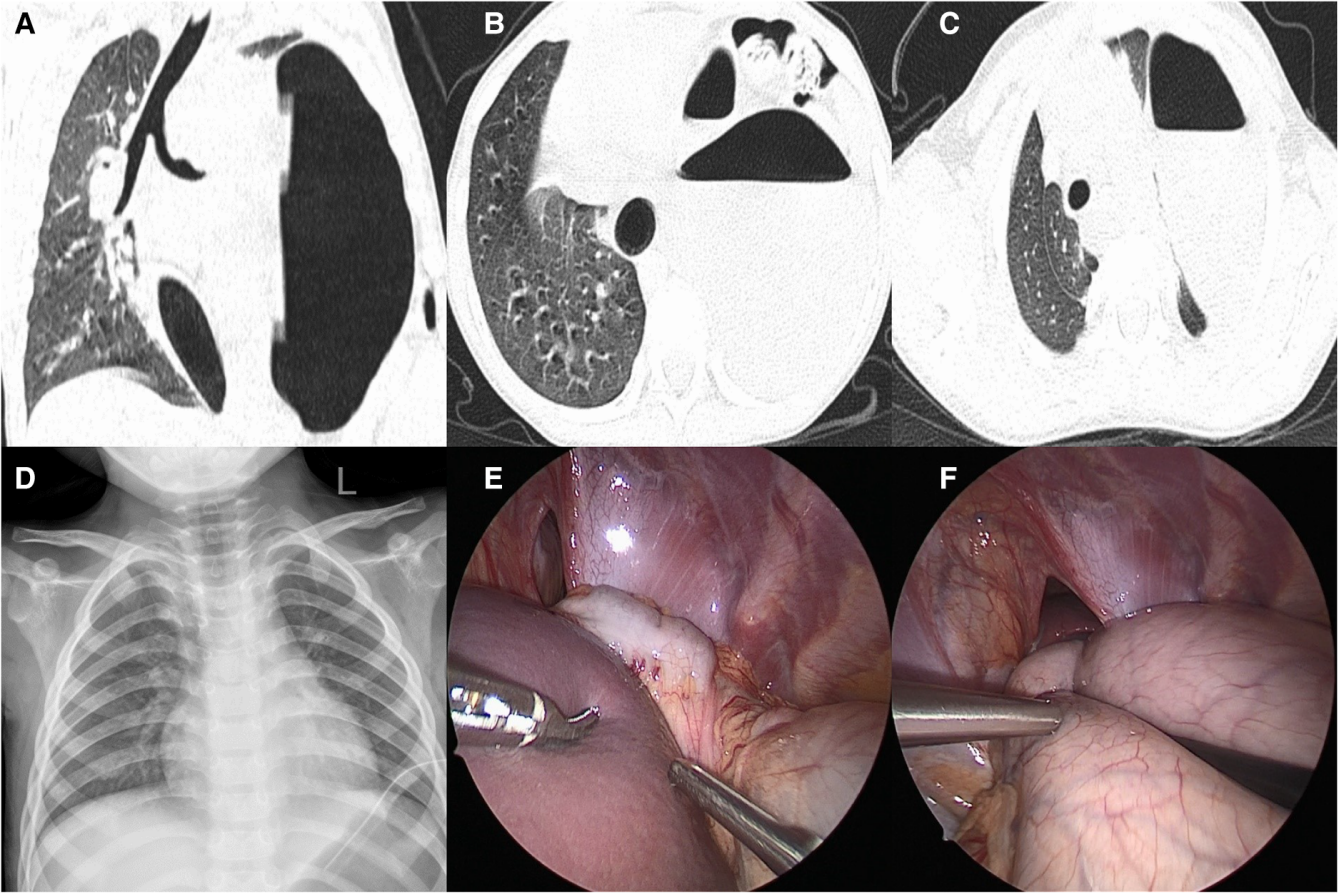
Case 2: preoperative CT, postoperative x-ray manifestations, and intraoperative conditions: (A) encapsulated gas accumulation with mediastinal displacement; (B) stomach and part of colon herniated into the thoracic cavity; (C) left lung was atelectasis and compressed to the upper of the stomach; (D) after the operation, the diaphragm recovered to normal position and the lung expanded well; (E) the hernia sac mouth is small, and the spleen and part of colon herniated into it; (F) the spleen had returned to the abdominal cavity and presented the hollow and herniated stomach in the thoracic cavity.
